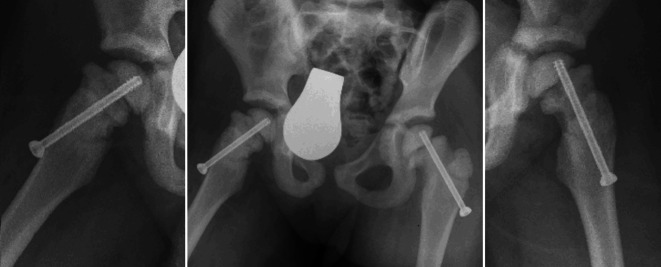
Postoperative anterior posterior and Lowenstein radiographs after osteosynthetic treatment of the bilateral femoral neck fractures
